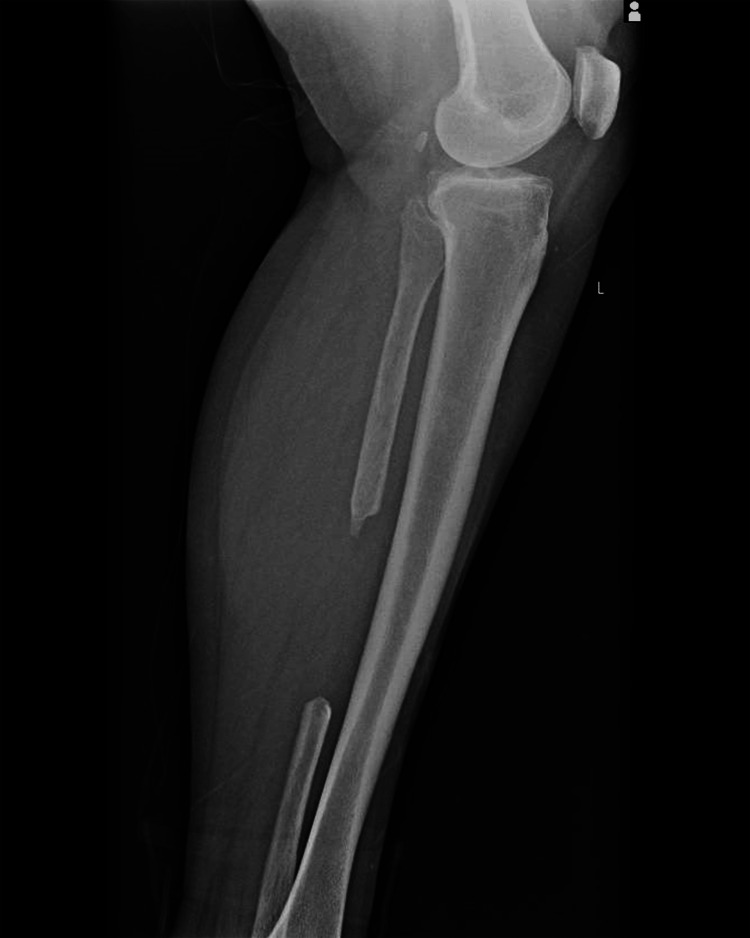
Lateral X-ray of the left leg taken two years postoperatively, with visible fibula shaft graft harvest site shown
